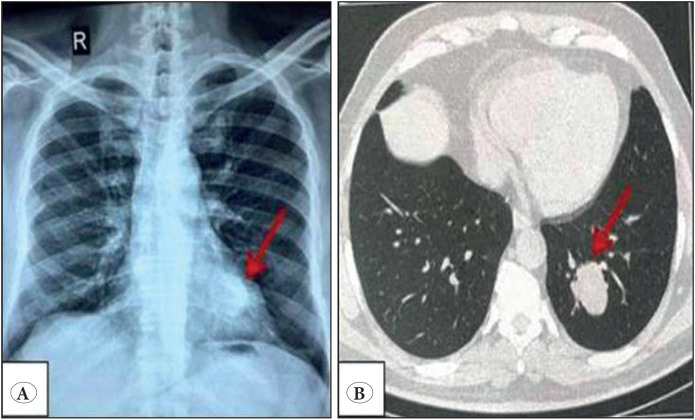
Chest X-ray (A) and CT scan (B) present a 33 mm, well-circumscribed, homogenous, non-calcified, solitary nodule in the left lower lobe.
